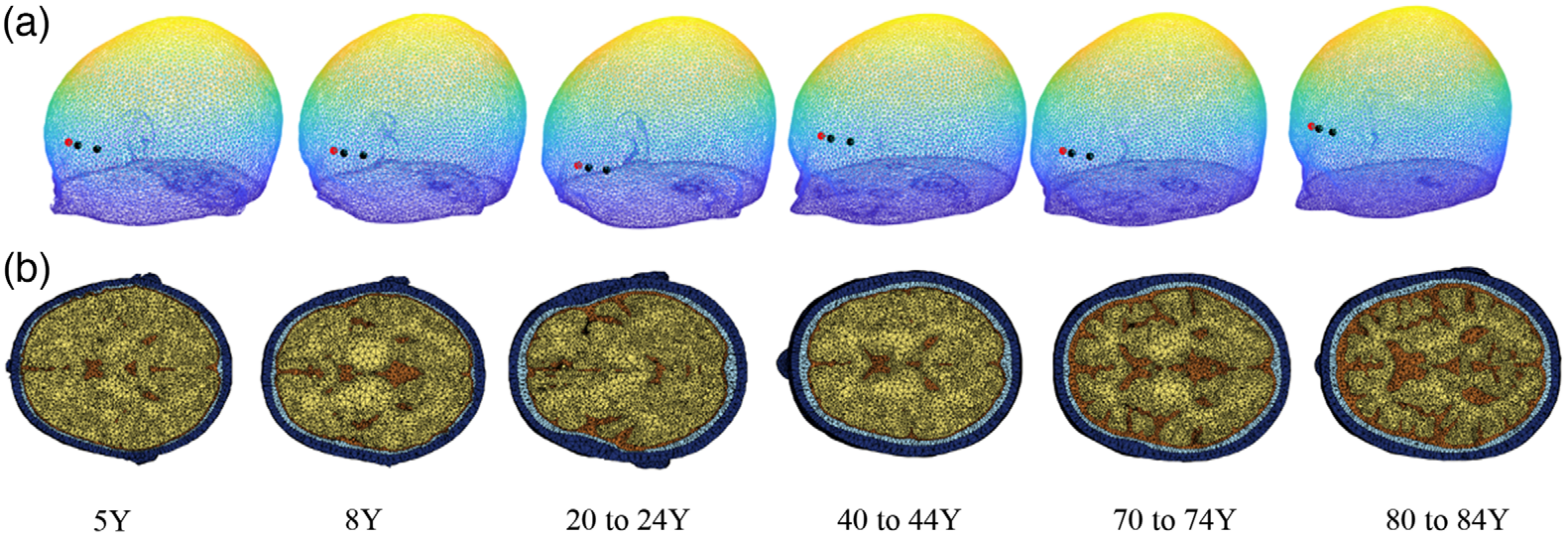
Age-averaged MRI templates. (a) 3D mesh of the contour of each atlas along with the source (red) and detectors locations (black). (b) Axial view of the plane where source and detectors were placed (scalp in dark blue, skull in light blue, CSF in brown, and brain in yellow).
